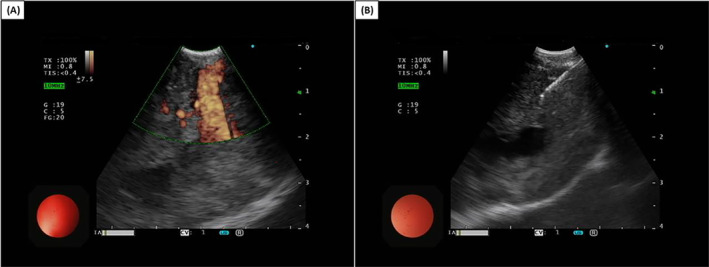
(A) An enlarged intrabronchial tumour under endobronchial ultrasound and (B) transbronchial needle aspiration biopsy.
